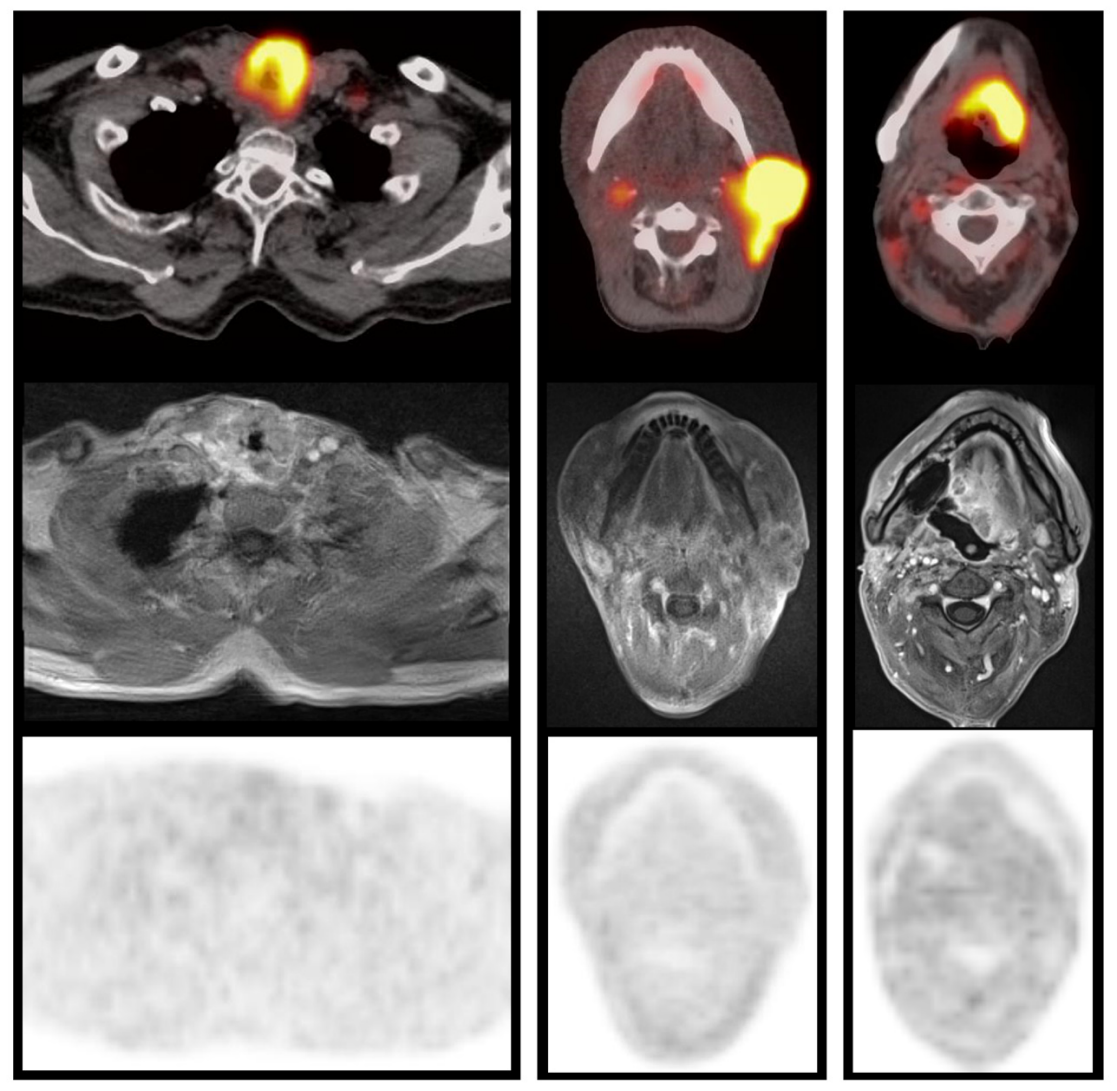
Patient examples. Examples of patients with local recurrence of a larynx cancer (left; patient #2), cervical lymph node metastasis (middle; patient #3) or oropharyngeal cancer relapse (right; patient #4), respectively. Fused FDG-PET/CT above, corresponding contrast-enhanced T1w MRI (StarVIBE) in the middle, and FMISO-PET below. In each patient, FDG uptake of the recurrent lesion is extensive and high, while specific FMISO uptake was absent (HV
